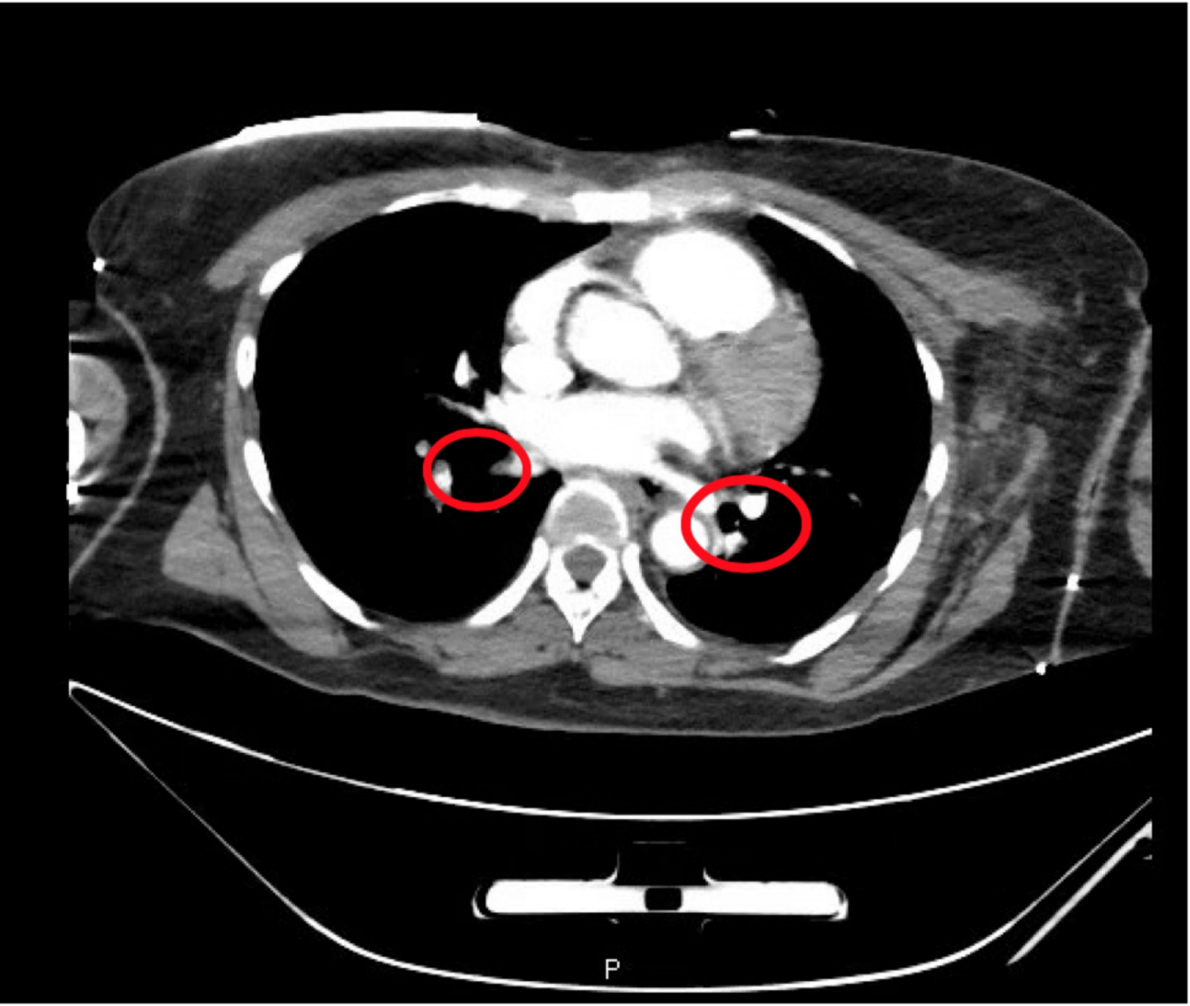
Computed tomography angiogram (CTA) with contrast demonstrates large bilateral subsegmental pulmonary embolisms in the truncus anterior and posterior basal segments
